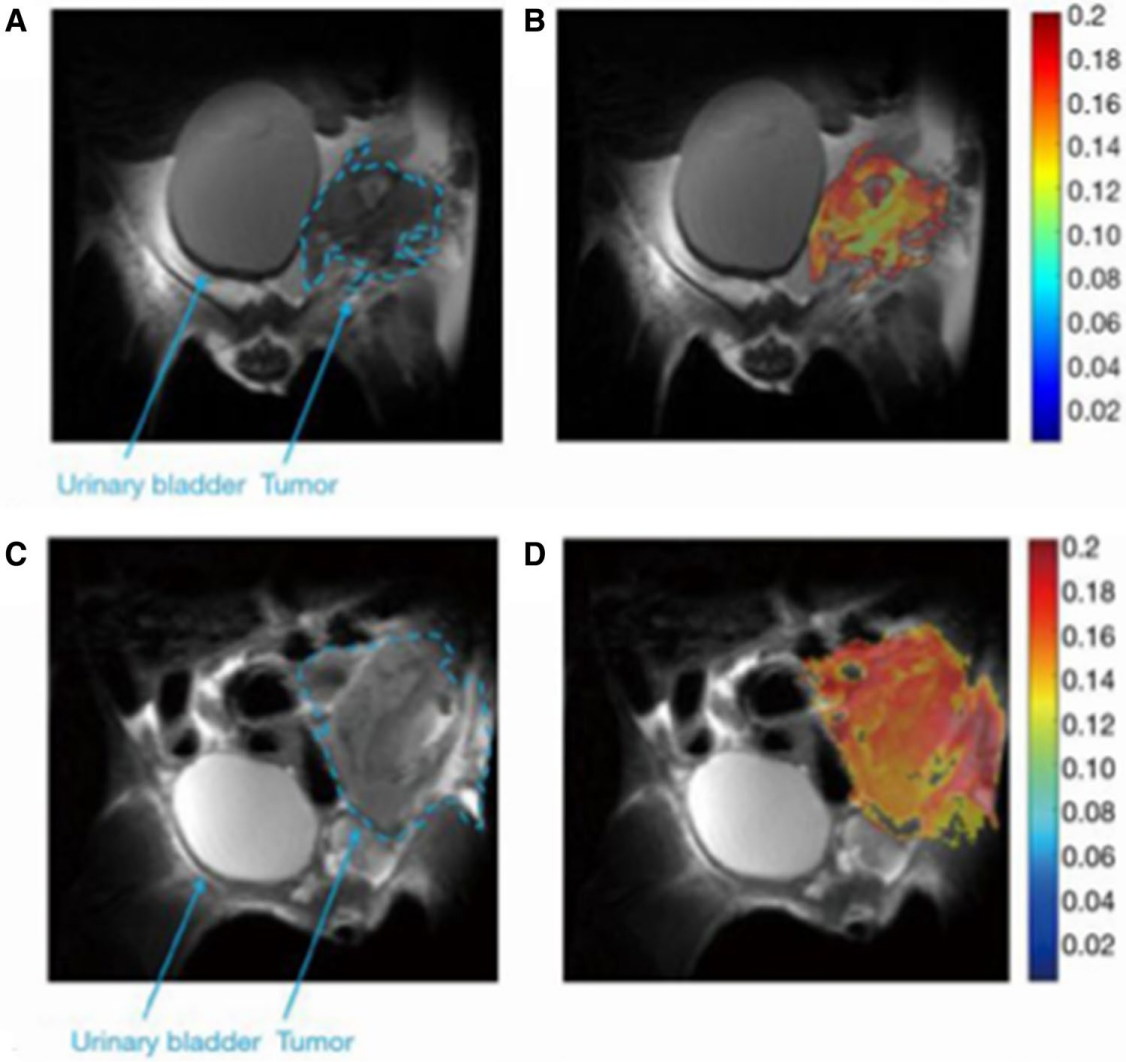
In vivo 2OMG and 6DG CEST MRI measurements in 4T1 tumors (7 T field). A, C T_2_-RARE anatomical images before administration of the agents. B, D MTR_asym_ images at 1.0 and 1.2 ppm following treatment with 2OMG (3 g/kg, IP) and 6DG (2.0 g/kg, IP), respectively, overlaid onto the T_2_ anatomical image. From [44], with permission
